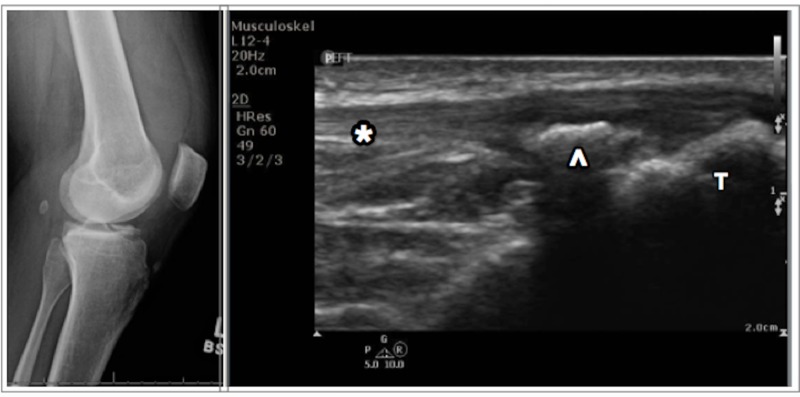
Radiograph of the left knee (left); ultrasound of the knee (right) demonstrating the tibia (T) with an avulsed portion of the anterior tibial spine (arrowhead), and an overlying intact patellar tendon (*)
